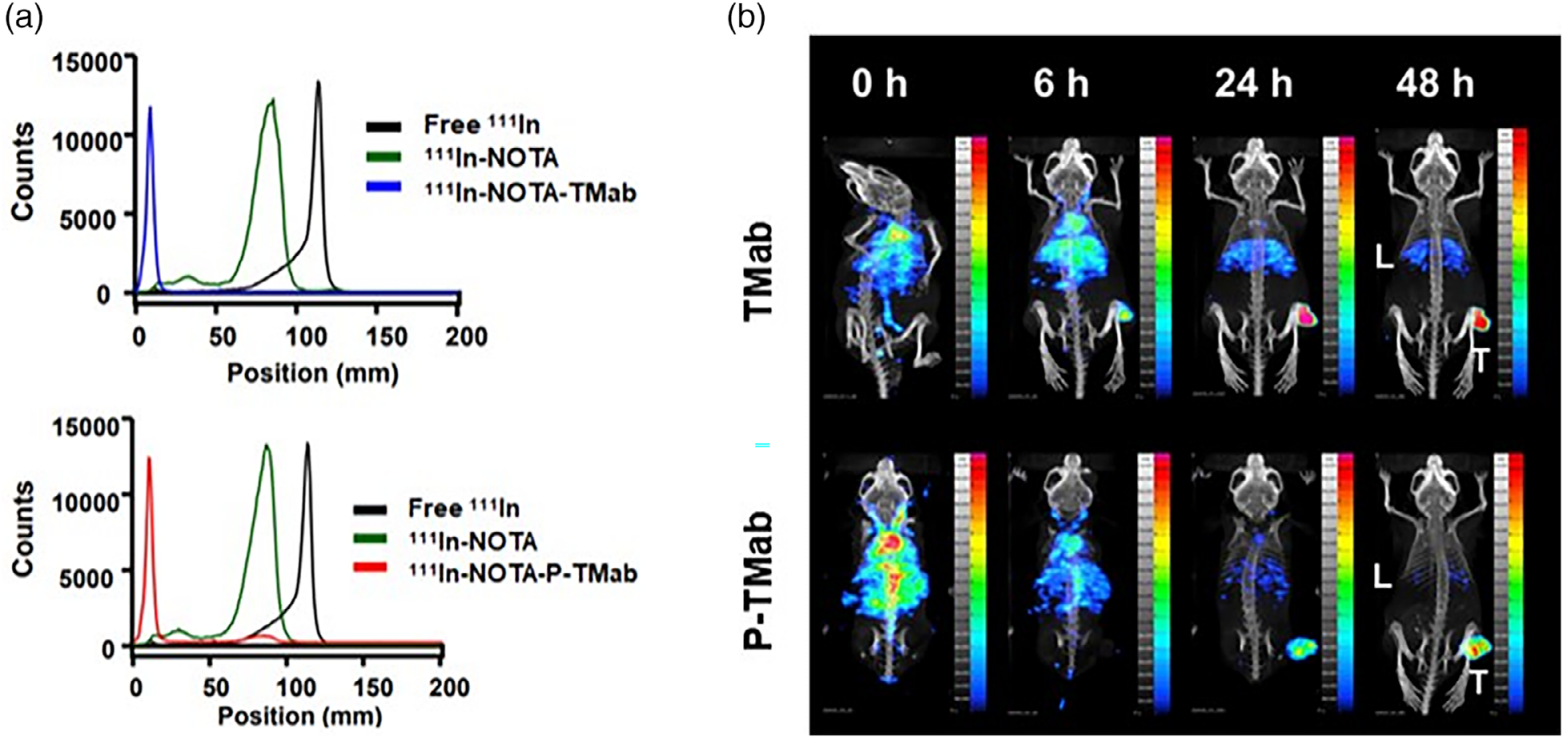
In vivo* biodistribution pattern of P‐TMab. (a) TLC results confirm antibody labelling efficiency of the radioactive isotope ^111^Indium. (b) SPECT/CT images of ^111^In‐labelled antibodies in BT474 tumour model. L and T indicate liver and tumour, respectively.
